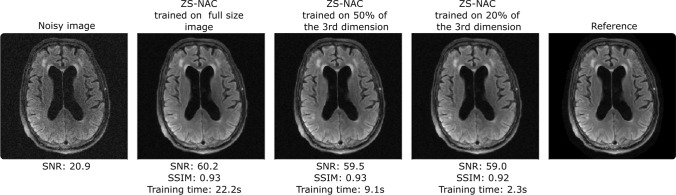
Examples of ZS-NAC denoising of fastMRI FLAIR data with an original matrix size = [320 × 320 × 16] using, respectively, 100%, 50% and 20% of the slices for training. All images are normalized to [0, 1] scale
